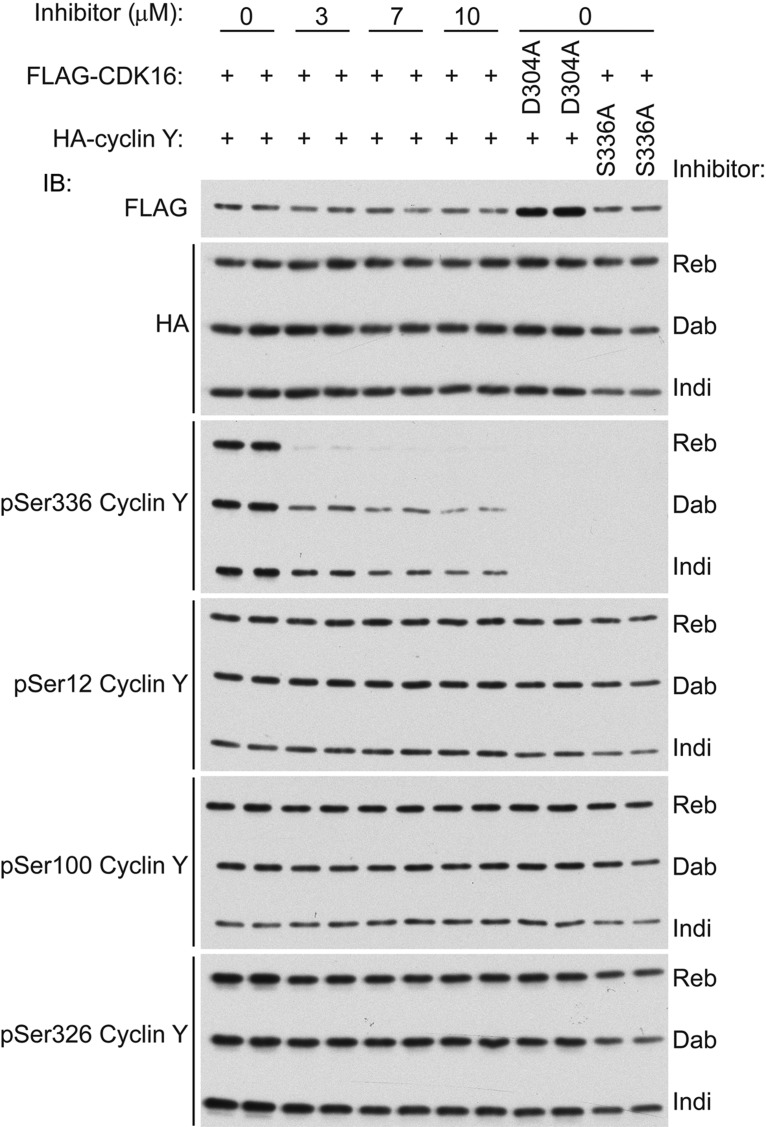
Cellular inhibition of CDK16. HA-cyclin Y WT or S336A mutant was co-transfected with FLAG-CDK16 WT or D304A kinase-inactive mutant in COS1 cells as indicated. Cells were treated for 1 h with varying concentrations of the indicated inhibitors, and cell lysates were immunoblotted using the indicated antibodies. FLAG immunoblot is representative of all three inhibitors. For other immunoblots (HA, pSer12, pSer100, pSer326 and pSer336), three separate blots (Reb, Dab and Indi) were exposed in the same X-ray film following incubation with enhanced chemiluminescence reagent. Reb, rebastinib; Dab, dabrafenib; Indi, indirubin E804.
